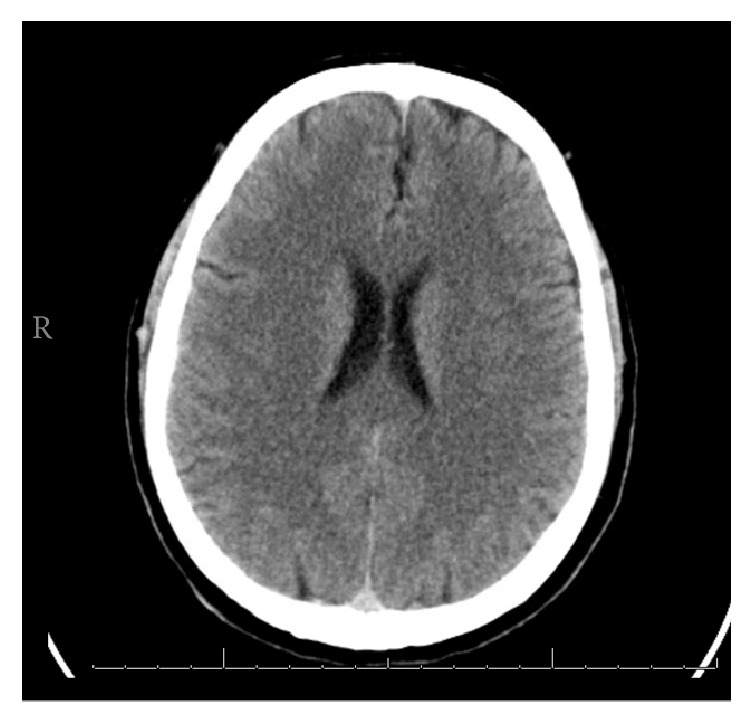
Case #2. Computed tomography of head without contrast with no acute abnormalities.
